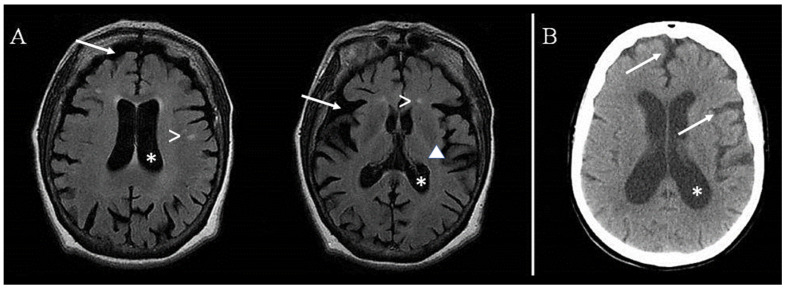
(A) Case 1: brain magnetic resonance imaging showing cerebral cortical atrophy, especially in the frontal lobes and perisilvian spaces (arrows), slight ventricular enlargement (asterisks), widening of the perivascular spaces (especially at the insular and nuclear-capsular levels, bilaterally) (▲), and mild signs of microangiopathy of the cerebral white matter (>). (B) Case 2: brain computed tomography scan showing diffuse cortical and subcortical atrophy (arrows), along with ectasia of the lateral ventricles (asterisk).
